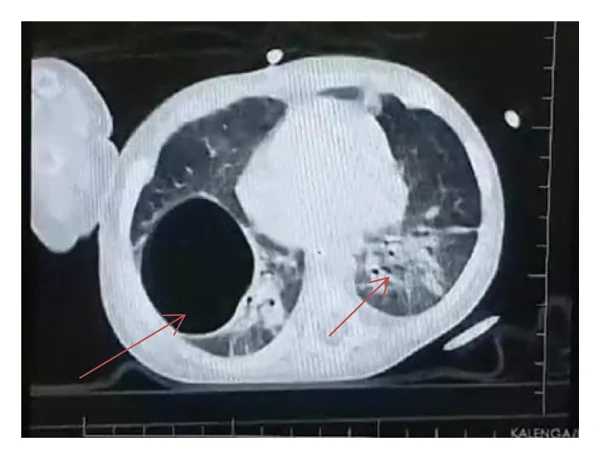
Axial view of CT scan of the chest showing a solitary large air‐filled cyst with consolidative changes in the lung fields.
